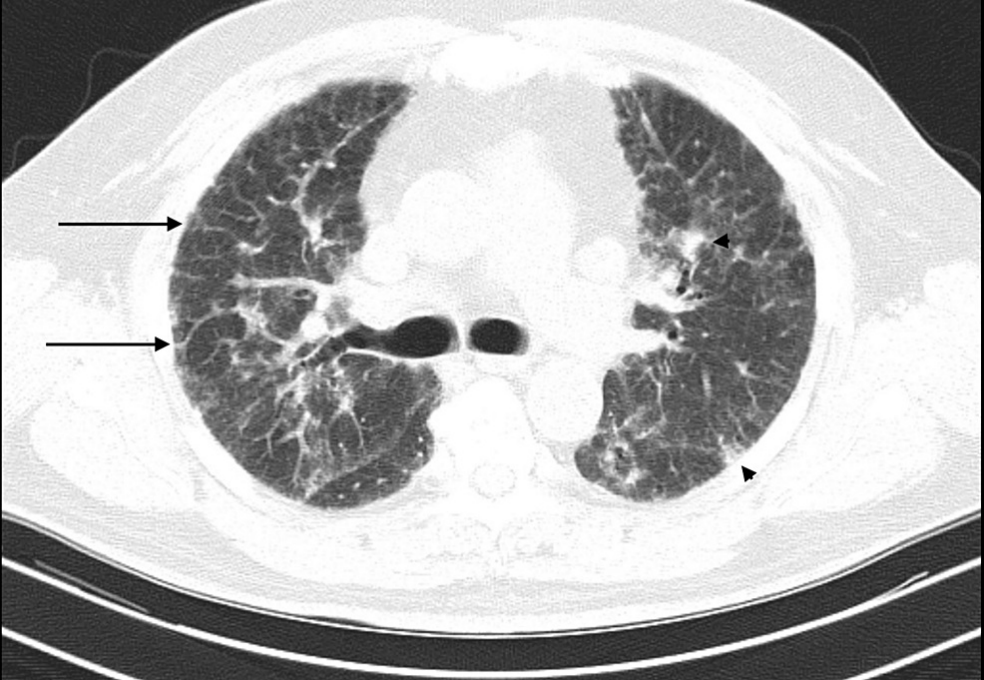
High-resolution computed tomography showing interstitial thickening with reticulation and ground-glass opacities Long arrows: reticulation; arrow heads: areas of ground-glass opacities
